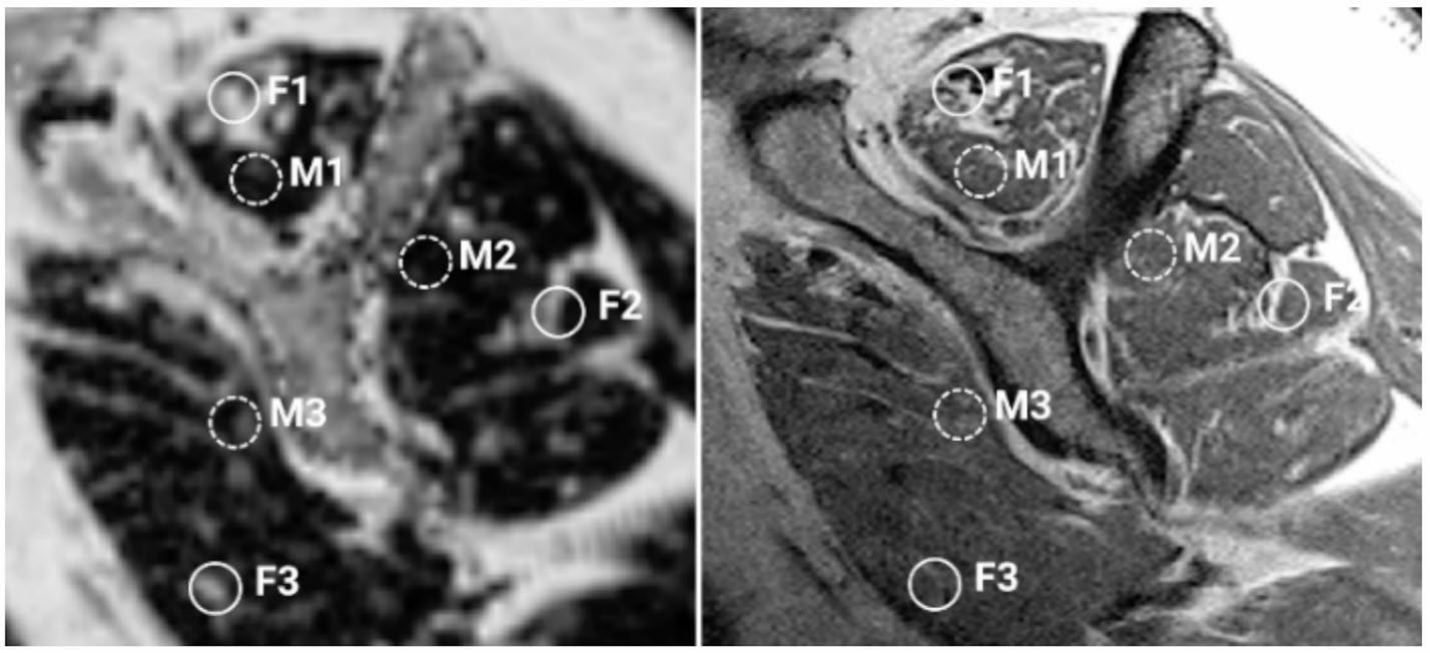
sagittal MRI from a patient with increased fat fraction of the RC muscles. Left: fat fraction image, right: T1-weighted MRI. Comparison of appearance in T1-weighted MRI and fat fraction image at the same location: all fatty streaks have similar fat fraction values; however, the T1-value differs: F1) T1: 291, F2) T1: 233 (80% of T1 of F1), F3) T1: 87 (30% of T1 of F1). Muscle tissues have similar values in T1-weighted MRI; however, they contain different amounts of fat fraction: M1) FF: 9% M2) FF: 10%/M3) FF: 30%.
